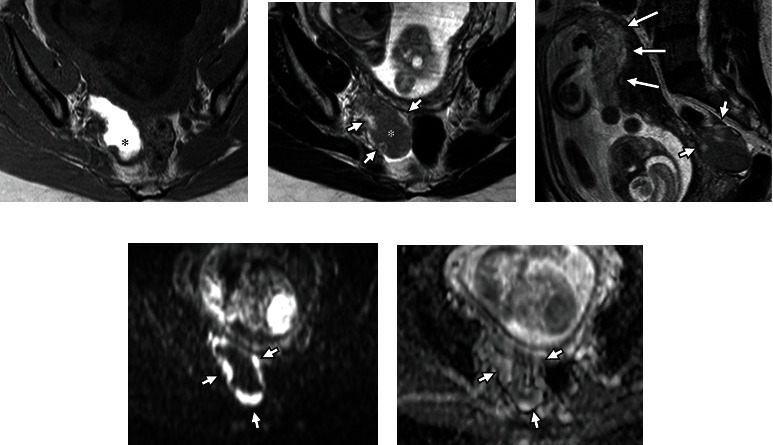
Magnetic resonance imaging (MRI) of the pelvis performed at 21 weeks of gestation. (a) Axial T1-weighted image. (b) Axial T2-weighted image. (c) Sagittal T2-weighted image. (d) Axial diffusion-weighted image (b factor: 1000 s/mm^2^). (e) Apparent diffusion coefficient (ADC) map. MRI shows a well-circumscribed teardrop-shaped cystic lesion with mural nodules measuring 68 × 45 × 35 mm in the right ovary. The cystic lesion shows uniformly marked high signal intensity on the T1-weighted image (a, asterisk) and intermediate signal intensity (b, asterisk) on T2-weighted images consistent with ovarian endometrioma. The mural nodules show intermediate to high signal intensity on T2-weighted images (b, c; short arrows) similar to the placenta (c, long arrows). Diffusion-weighted image (d, short arrows) and ADC map (e, short arrows) show the mural nodules with a high ADC value (1.6 × 10^−3^ mm^2^/s). Based on these imaging findings, we diagnosed a decidualized ovarian endometrioma.
